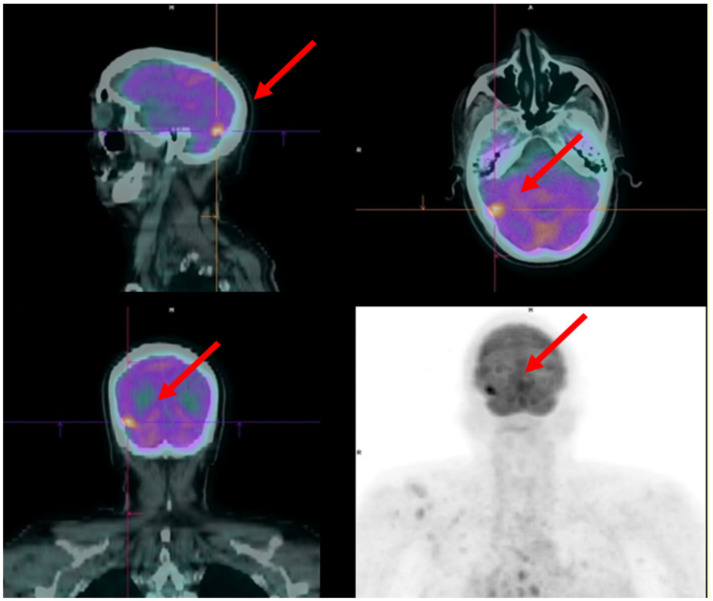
Brain metastasis in the right temporo-basal cortex (red arrows) incidentally discovered by 18F-FDG PET in an mCRPC patient candidate to the Ra-223 therapy.
